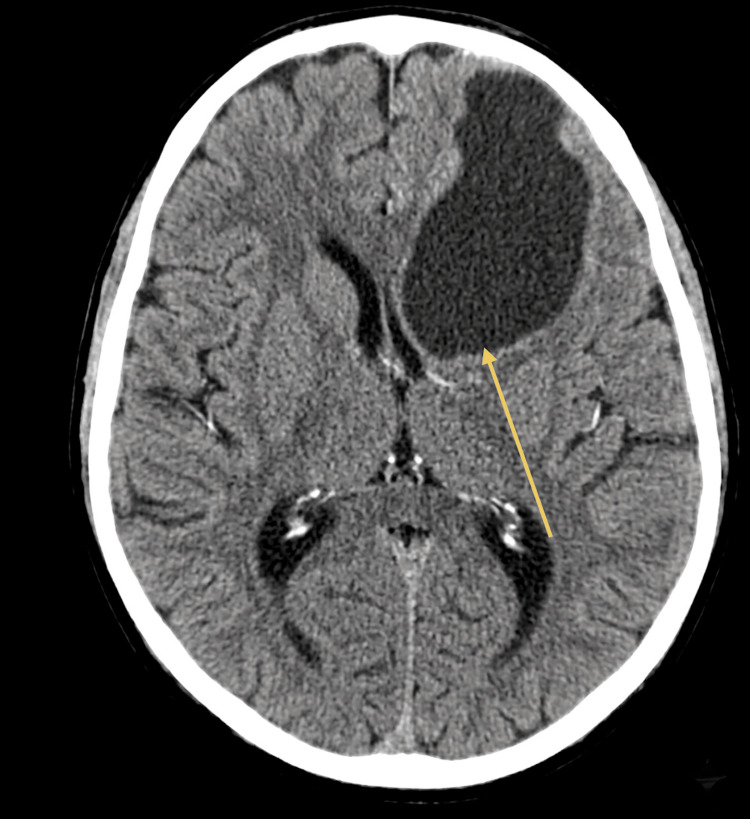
Non-contrast MRI of the brain showed a solid-cystic mass at the genu of the corpus callosum The yellow arrow points to the solid cystic mass lesion margin adjacent to the left lobe, compressing the nearby ventricle and midline shift.
